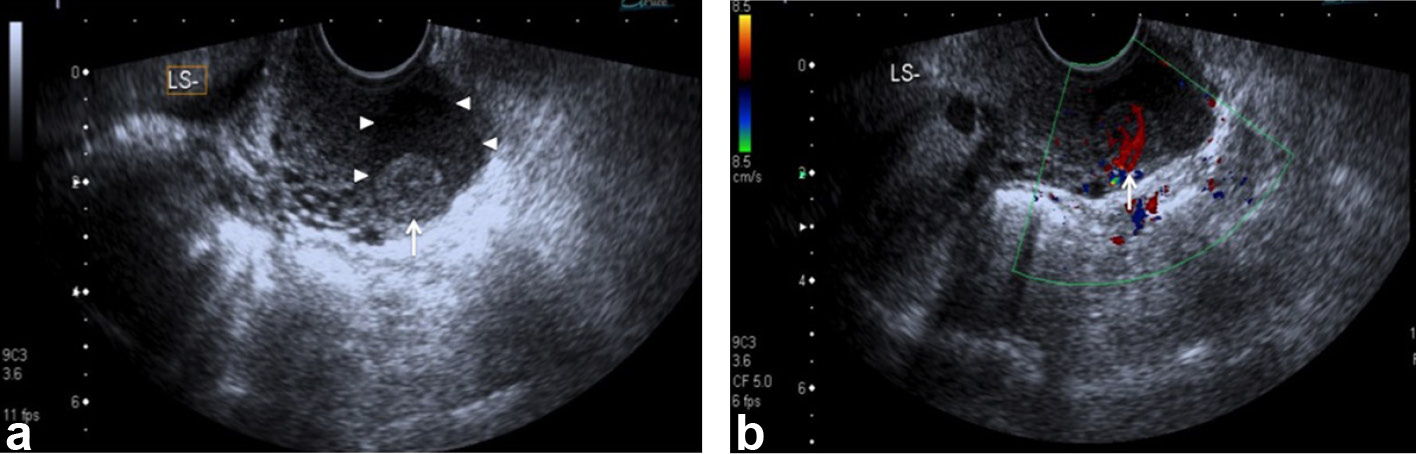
(a and b) Transvaginal B-mode ultrasound demonstrates a 25 mm cystic lesion
